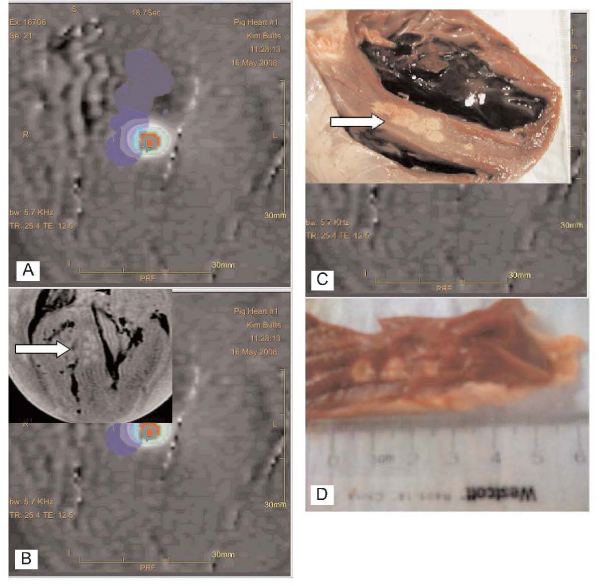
A) Real-time MR-temperature map during HIFU septal ablation (red zone on image), B) T2 MR image of HIFU lesions, C) Gross pathology of septal lesions from (B) using multiple 20 s pulses, D) Lesions, each 4 mm × 4 mm created with single 20 s HIFU pulses along the LV lateral wall.
